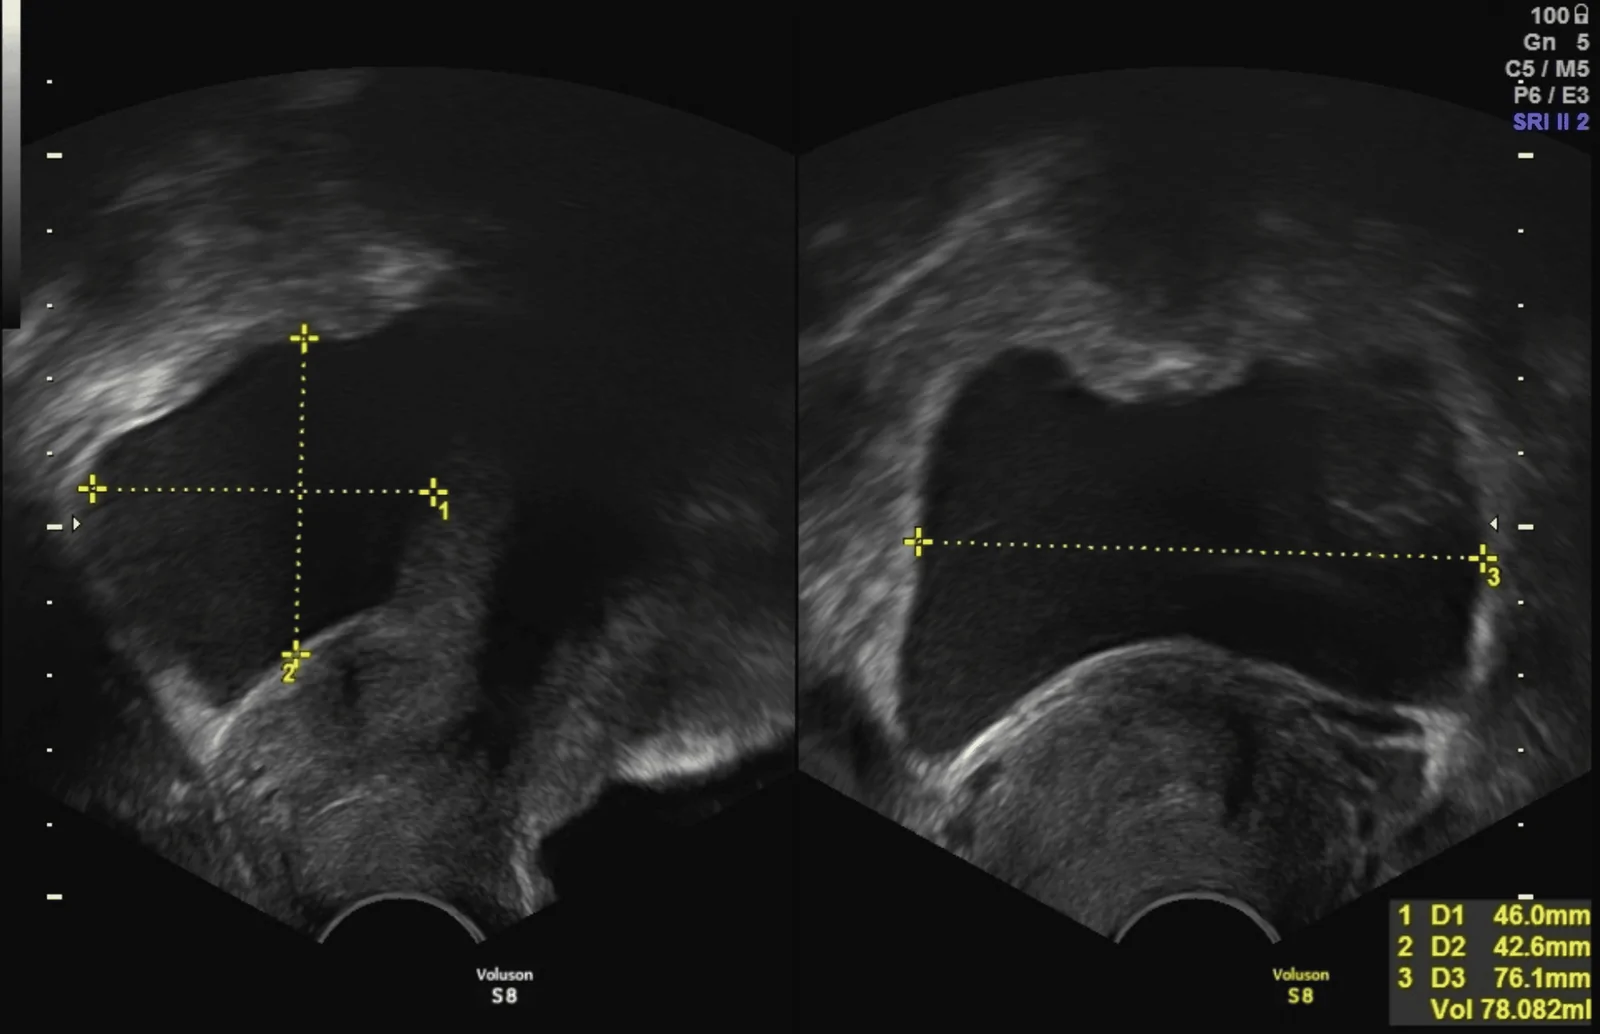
Transvaginal ultrasonography showing free intraperitoneal fluid consistent with hemoperitoneum.
